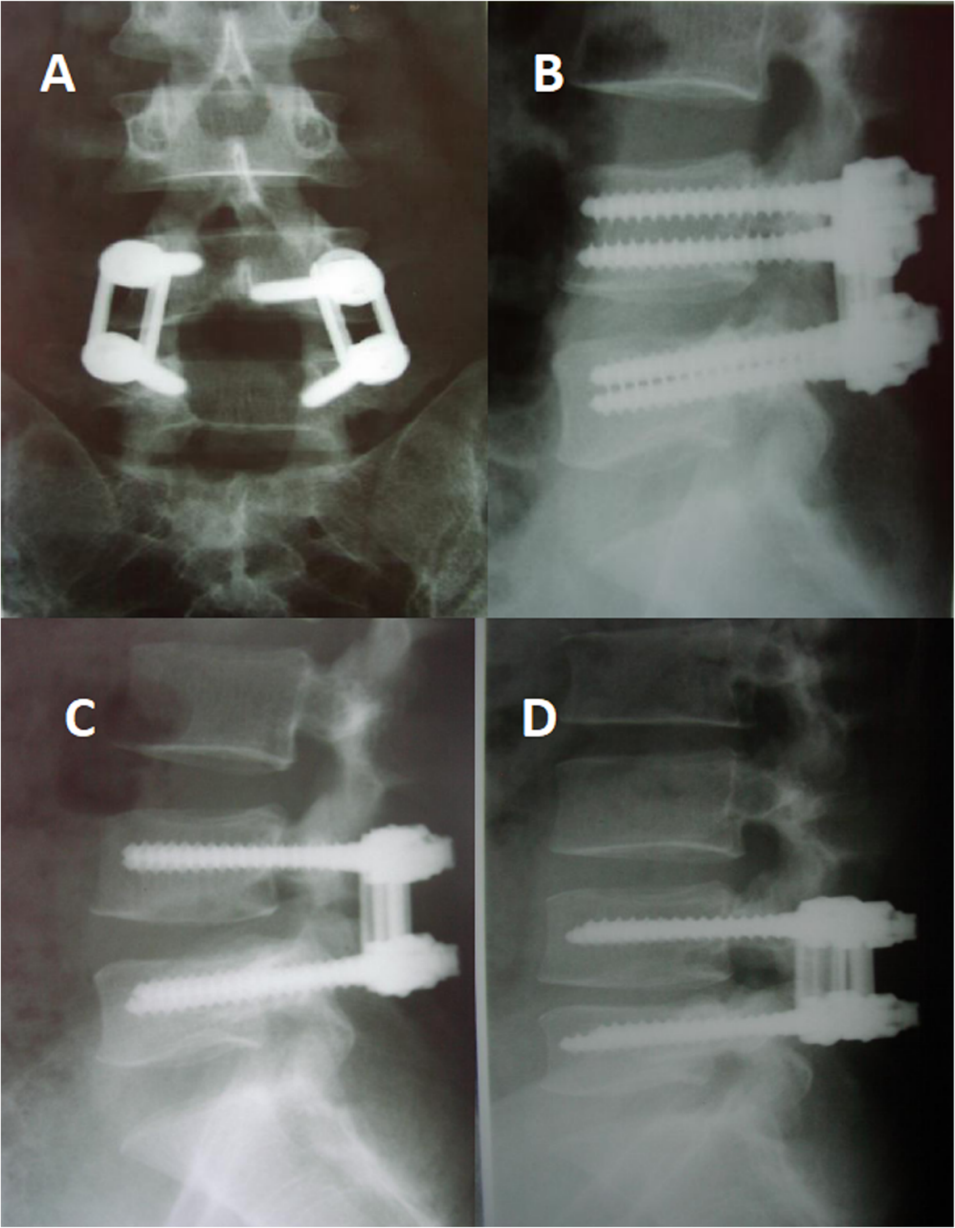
L4 DLS in a patient treated with L4 laminectomy and posterolateral fusion. Because of leg pain, she underwent revision surgery (ab). Postoperative X-ray showed screw displacement. c Postoperative X-ray showed that the screw was corrected and the pain improved. d L4-L5 disc height narrowing and posterolateral fusion were observed at the 48-month follow-up
